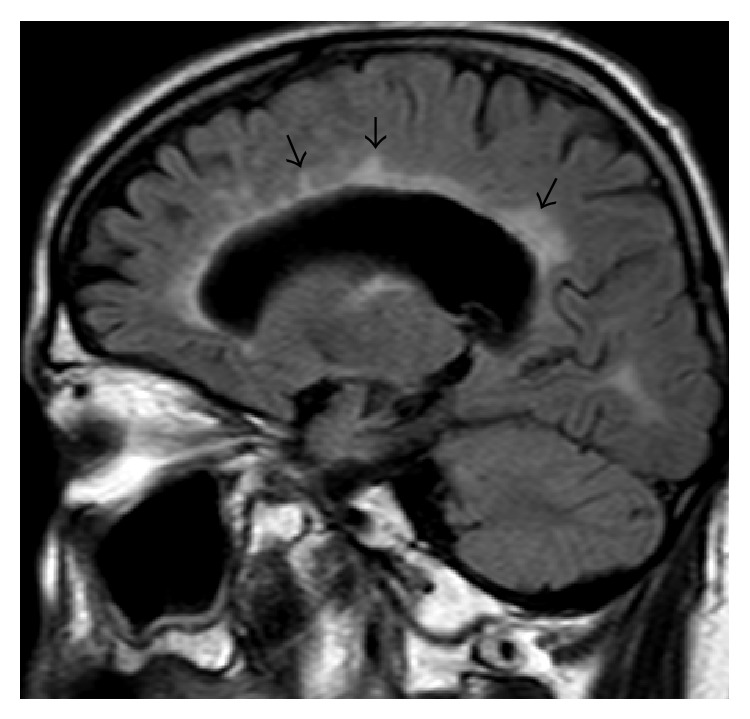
Left parasagittal FLAIR image of the brain of the patient at the time of his relapse (March 2012). Multiple bright white matter lesions with the long axis perpendicular to the surface of the lateral ventricle compatible with multiple sclerosis.
